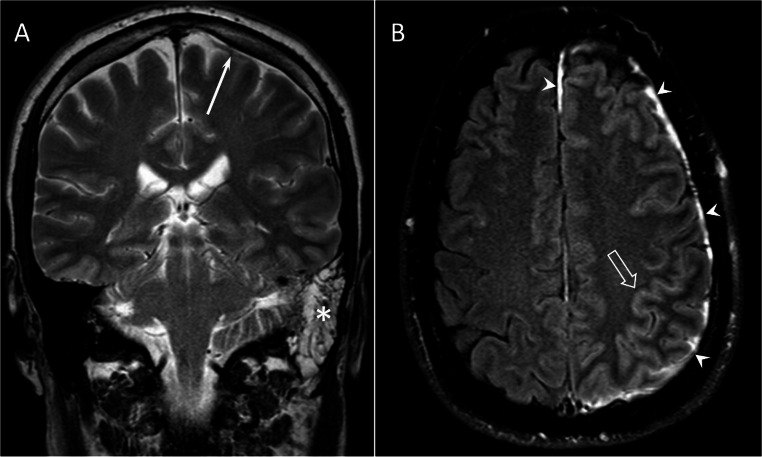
Otogenic meningitis on MRI. Obliteration of the left mastoid (asterisk) and meningeal thickening (arrow) on coronal T2 (A). Enhancement of the dura (arrowheads) and cerebral sulci (open arrow) on axial Gd FLAIR (B)
